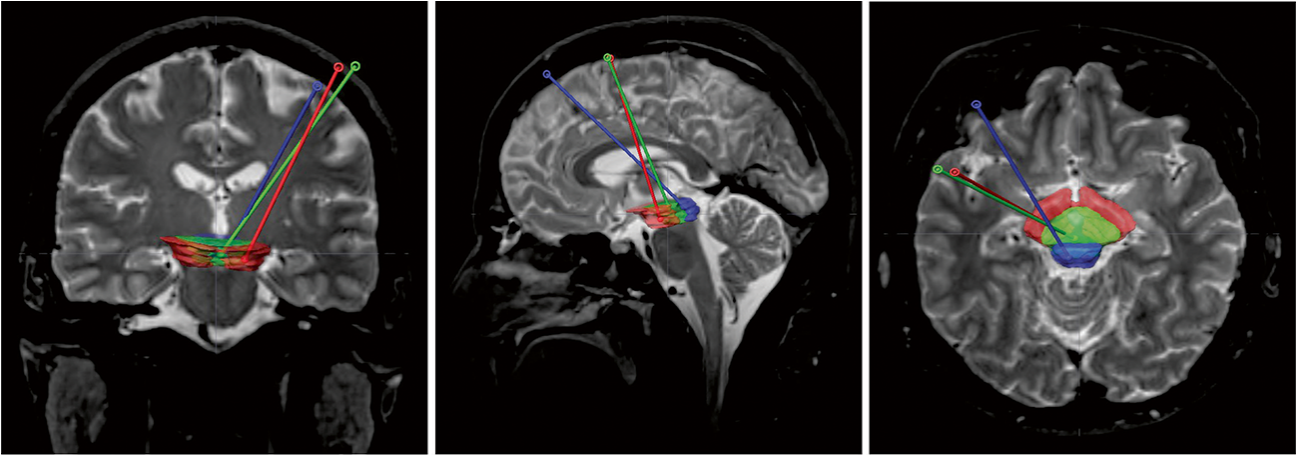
Coronal (left), sagittal (middle), and axial (right) T2-weighted MRI images showing the proposed midbrain regions of interest (ROI) and the preferred trajectory (red, crus cerebri; green, tegmentum; blue, tectum)Lesions in the pons accessible through an infratentorial, transcerebellar-transpeduncular approach should preferentially be targeted by this approach due to the short trajectory and paucity of any eloquent tracts or nuclei along this trajectory. Superior pontine lesions as well as lesions in the midline of the pons are often not accessible through a transcerebellar-transpeduncular trajectory and a supratentorial transfrontal approach can be performed in these cases (Fig. 5).
